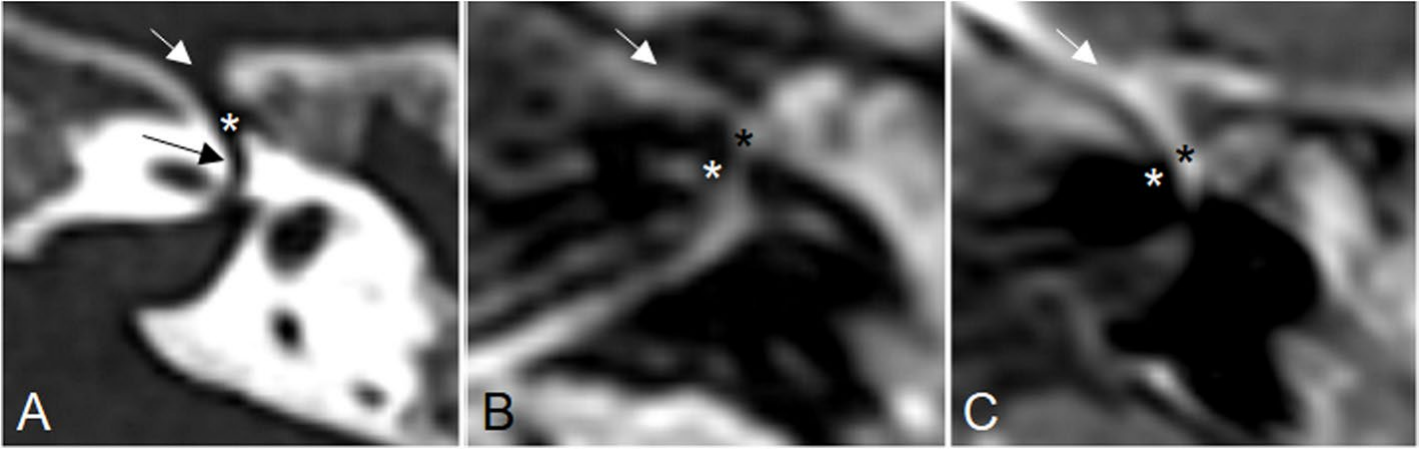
Left temporal bone (A) CT and (B, C) MR axial plane images. A The CT image is showed for anatomic reference (black arrow: bony canal of the labyrinthine segment of the facial nerve; white arrow: bony canal of the greater petrosal nerve; asterisk: geniculate ganglion fossa). The MR images show (B) slight FLAIR hyperintensity and subtle (C) T1 contrast enhancement of the geniculate ganglion (black asterisk), labyrinthine portion of facial nerve (white asterisk), and proximal tract of the greater petrosal nerve (white arrow)
